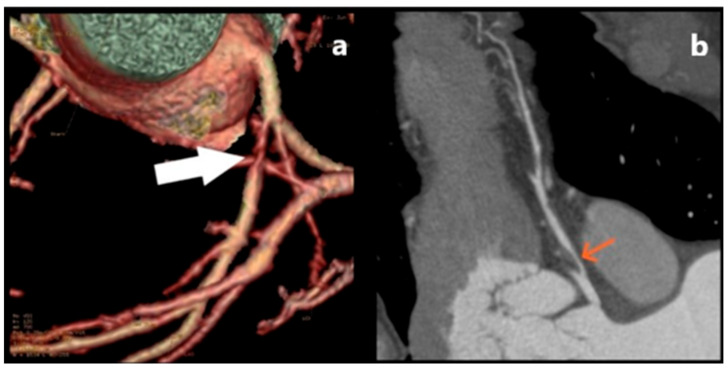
MD CT coronary angiography showing (a) three-dimensional-volume rendered image showing a segmental stenosis of the left anterior descending artery—LAD (white arrow)—as well as a (b) plane image of the stenosis (orange arrow). With noted high-grade stenosis of the proximal segment of the LAD secondary to vasculitis, the patient experienced severe anginal symptoms. In addition to optimal medical therapy, including beta-blockers, nitrates, and calcium channel blockers, surgical revascularization by coronary artery bypass graft (CABG) was indicated.
